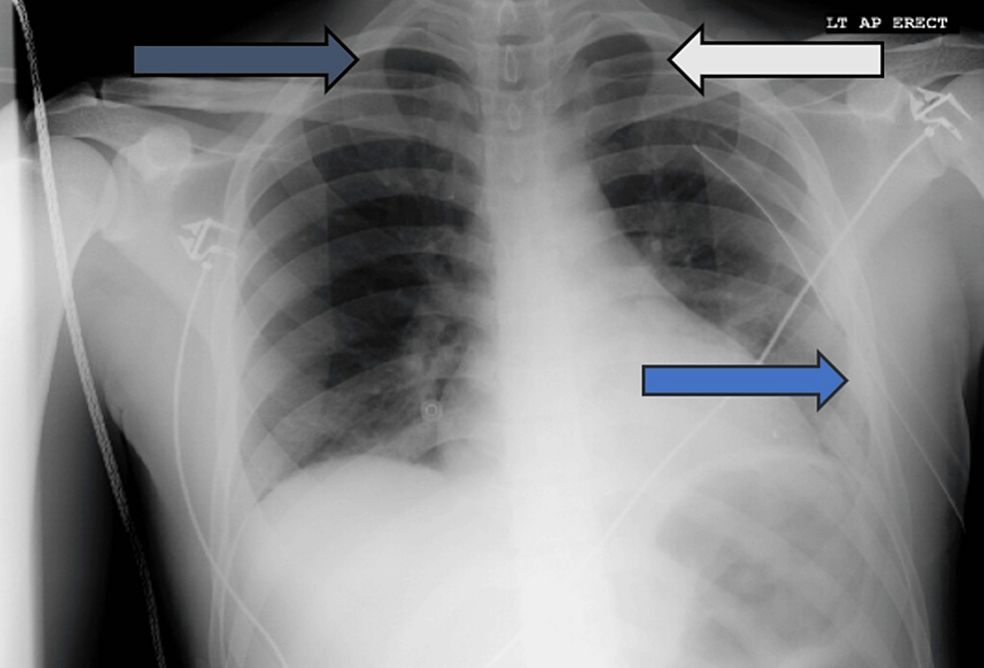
Chest X-ray done on post-operative day 8. The blue arrow indicates the chest tube inserted on presentation to the emergency department is still in the correct position. The white arrow indicates a clear left lung field with no associated pneumothorax or hemothorax. The gray arrow indicates a clear right lung field with no associated pneumothorax or hemothorax.
